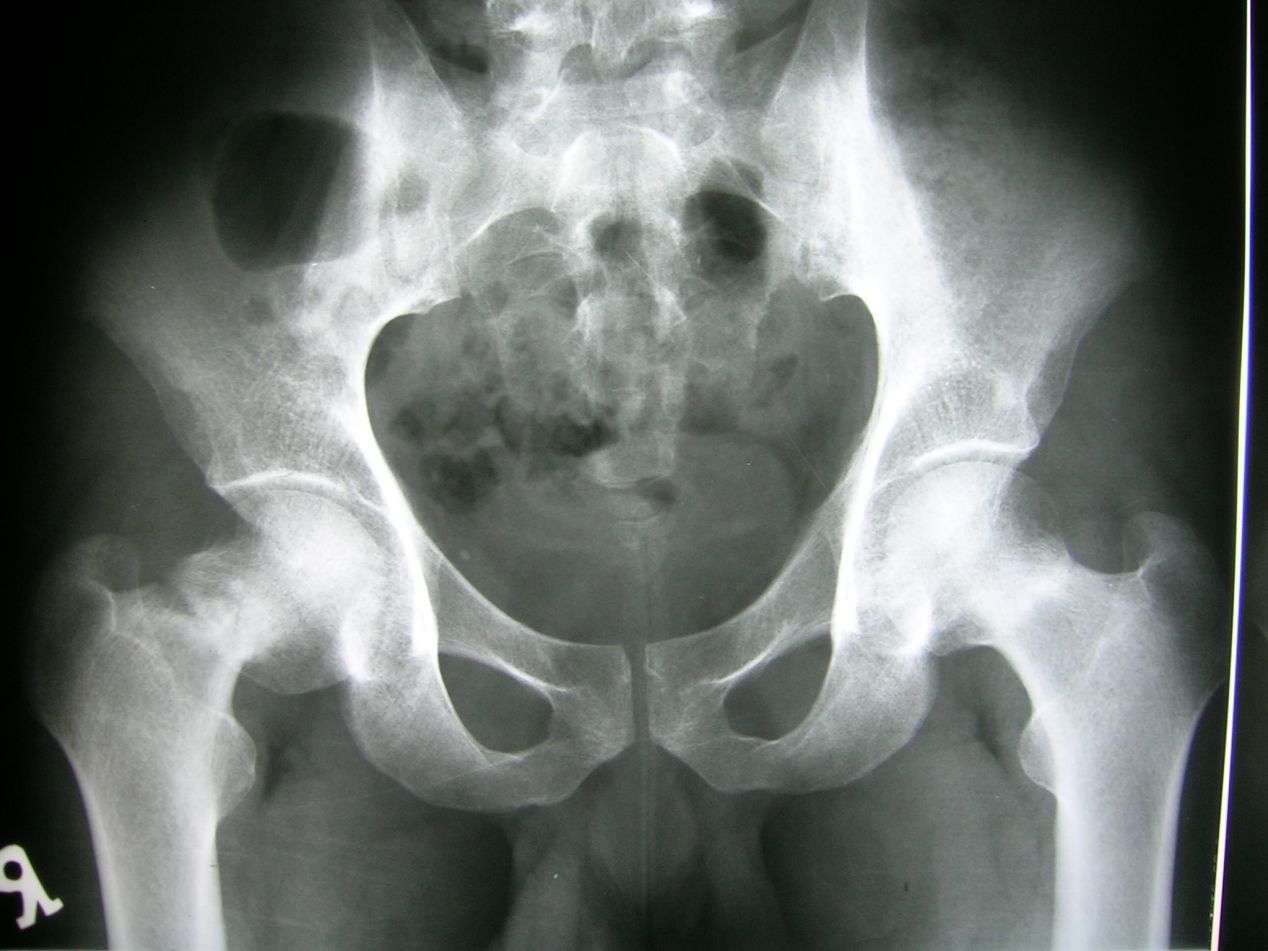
Radiograph taken 4 months before operation demonstrates displaced left femoral neck stress fracture and non-displaced right femoral neck stress fracture.
